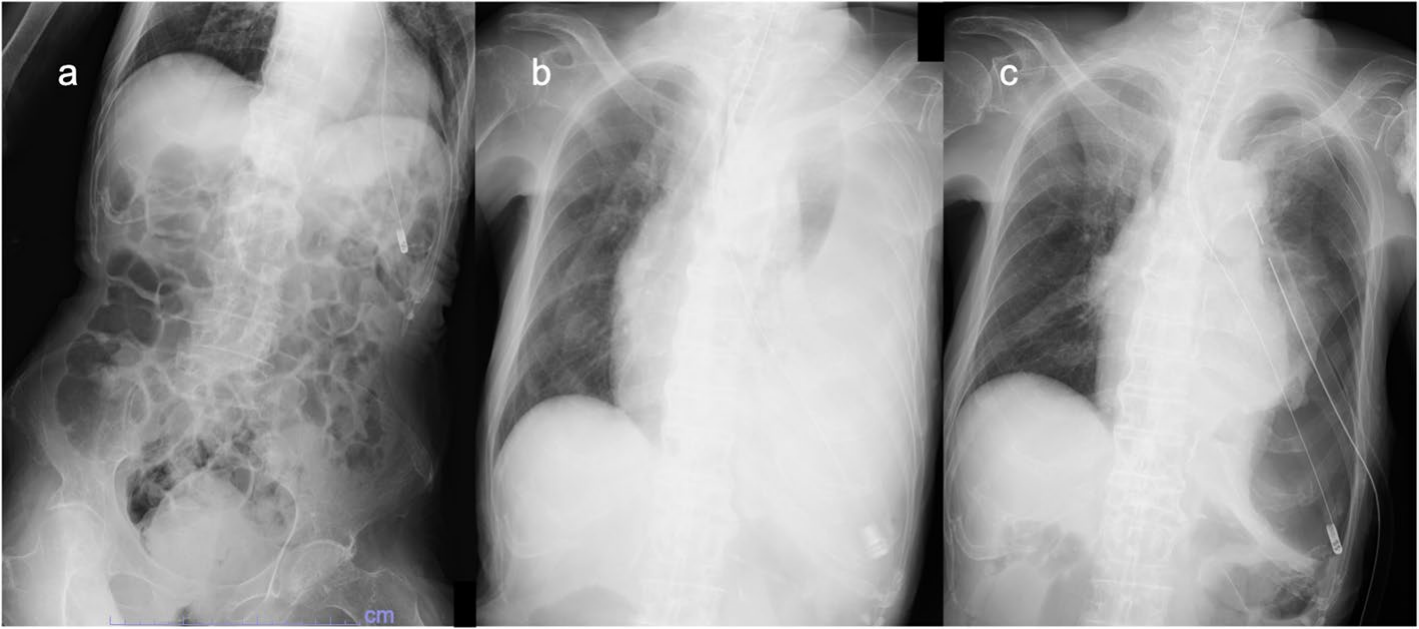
Chest X-ray images. a Immediately after the nasogastric tube (NGT) replacement. b Decreased radiolucency in the entire left lung field 19 h after the NGT replacement. c Misinserted NGT also shown after pleural space drainage at our department
